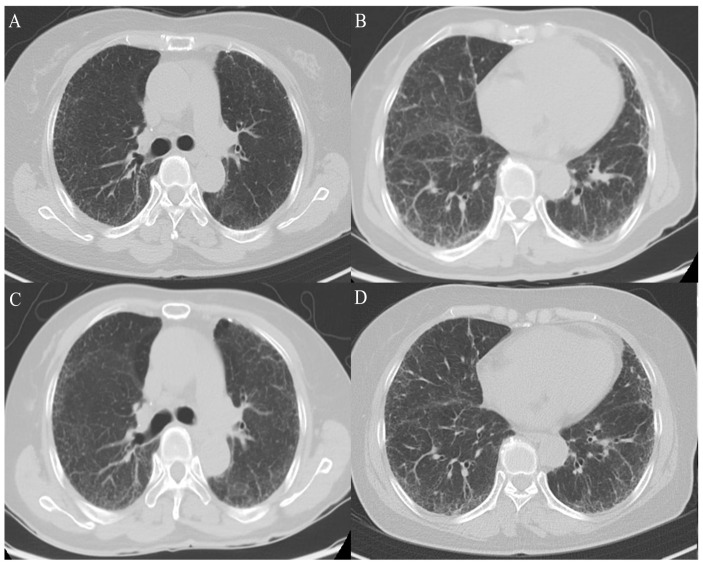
Disease status of IPF over time. A comparison between CT images from 6 October 2022 (A,B) and 17 October 2023 (C,D) shows no significant differences. IPF, idiopathic pulmonary fibrosis; CT, computed tomography.
